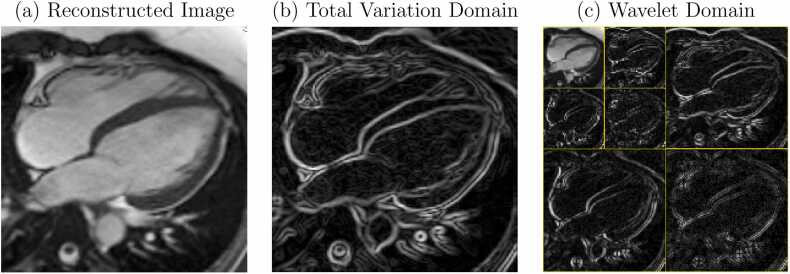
Sparse domains for CS reconstruction. The reconstructed MR image in (a) has sparse representations in the total variation (b) and wavelet (c) domains as only a small fraction of the total coefficients in these domains are non-zero. *CS* compressed sensing, *MR* magnetic resonance
